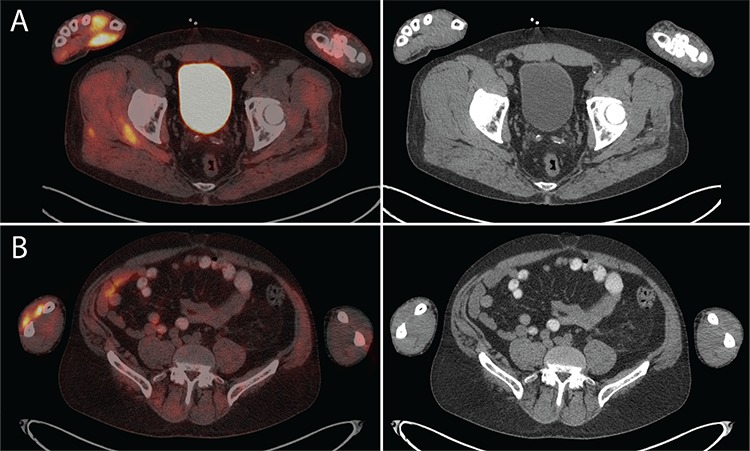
(A) Intense ^18^F-FDG uptake in the right thenar eminence with maximum standard uptake value (SUV_max_) 8.8 was the most intensely ^18^F-FDG avid abnormality in the entire PET/CT study, and was most likely the result of the patient holding beer cans and drinking beer. (B) Right dorsal extensor forearm muscles also showed intense ^18^F-FDG uptake with SUV_max_ 6.7.
